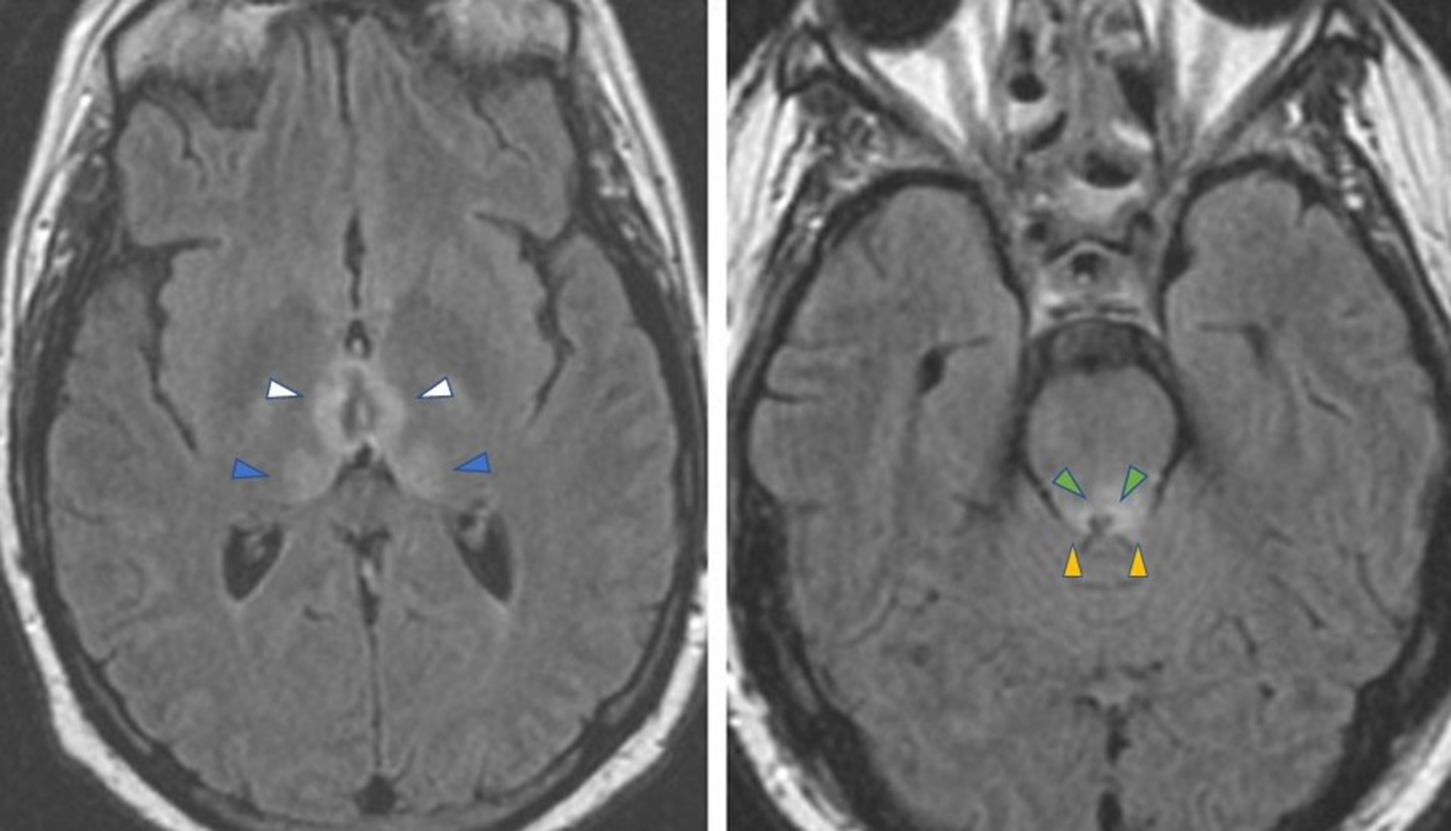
Axial T2-FLAIR images in a patient with acute WE are shown. Typical MRI findings in acute WE include T2 prolongation along the medial thalami (blue arrowhead) and mammillothalamic tracts (white arrowheads) on the left image, as well as the periaqueductal gray (green arrowheads) and tectal plate (yellow arrowheads) on the right image.
